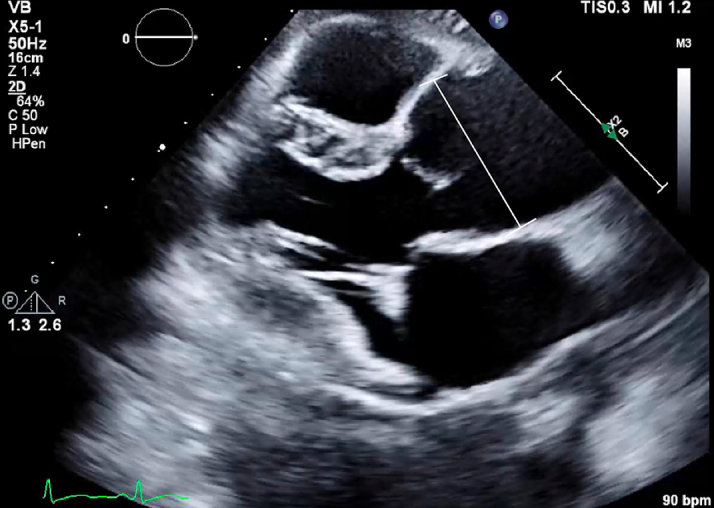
Preoperative Transthoracic Echocardiogram Demonstrating a Dilated Aortic Root to 4.1 cm
